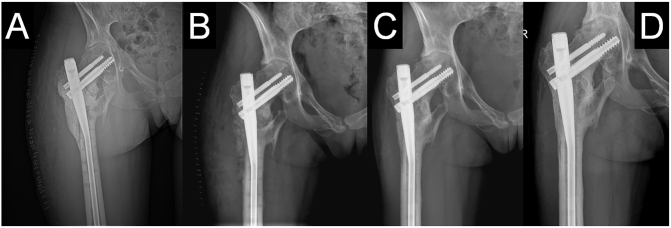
Anteroposterior (AP) pelvic radiographs showing bone defect filled with PMMA cement spacer after stage one operation (A) and graft integration immediately (B), 3 months (B), 6 months (C) and 11 months (D) after stage two Masquelet operation.
